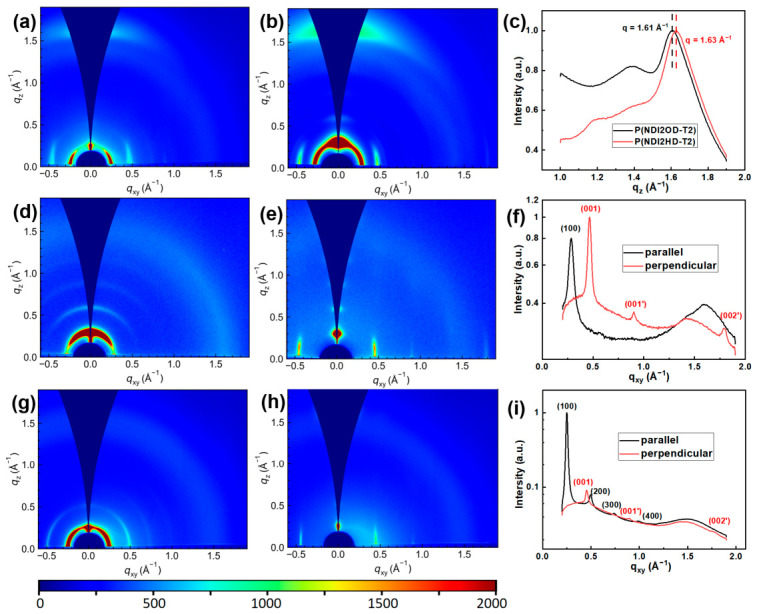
The 2D-GIWAXS images of the P(NDI2OD-T2) (a,g,h) and P(NDI2HD-T2) (b,d,e) films grown via spin-coating (a,b) and SVA-HMF (d,e,g,h). The X-ray beam is parallel (d,g) and perpendicular (e,h) to the HMF direction. (c) Cross-section profiles along the out-of-plane orientation of the 2D-GIWAXS patterns shown in (a,b); (f,i) are the cross-section profiles along the in-plane orientation of the (d,e) and (g,h), respectively.
